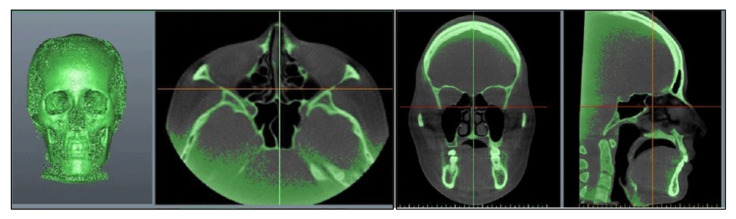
Reconstructing the 3D bone structure by importing 3D CBCT images.
